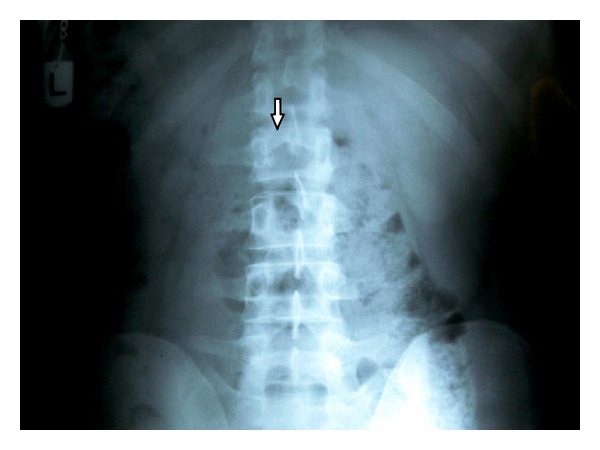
Abdominal radiographic image: presence of orthodontic wire in stomach location.
